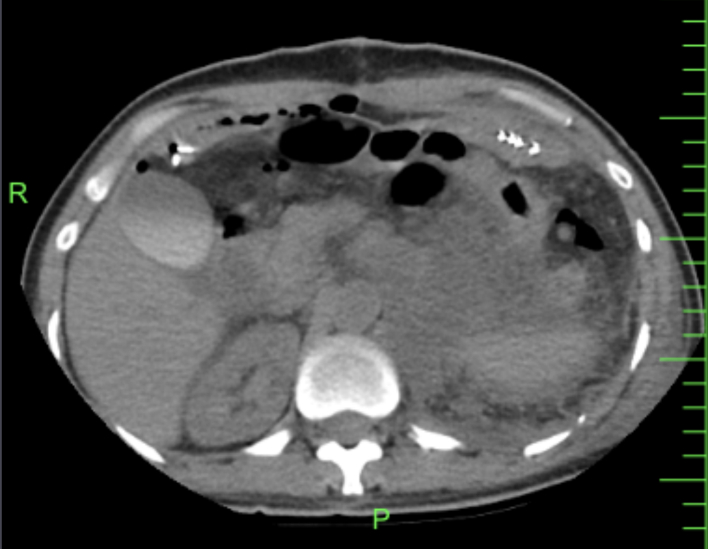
Follow-up CT scan images after the second laparotomy showing no residual mass.
